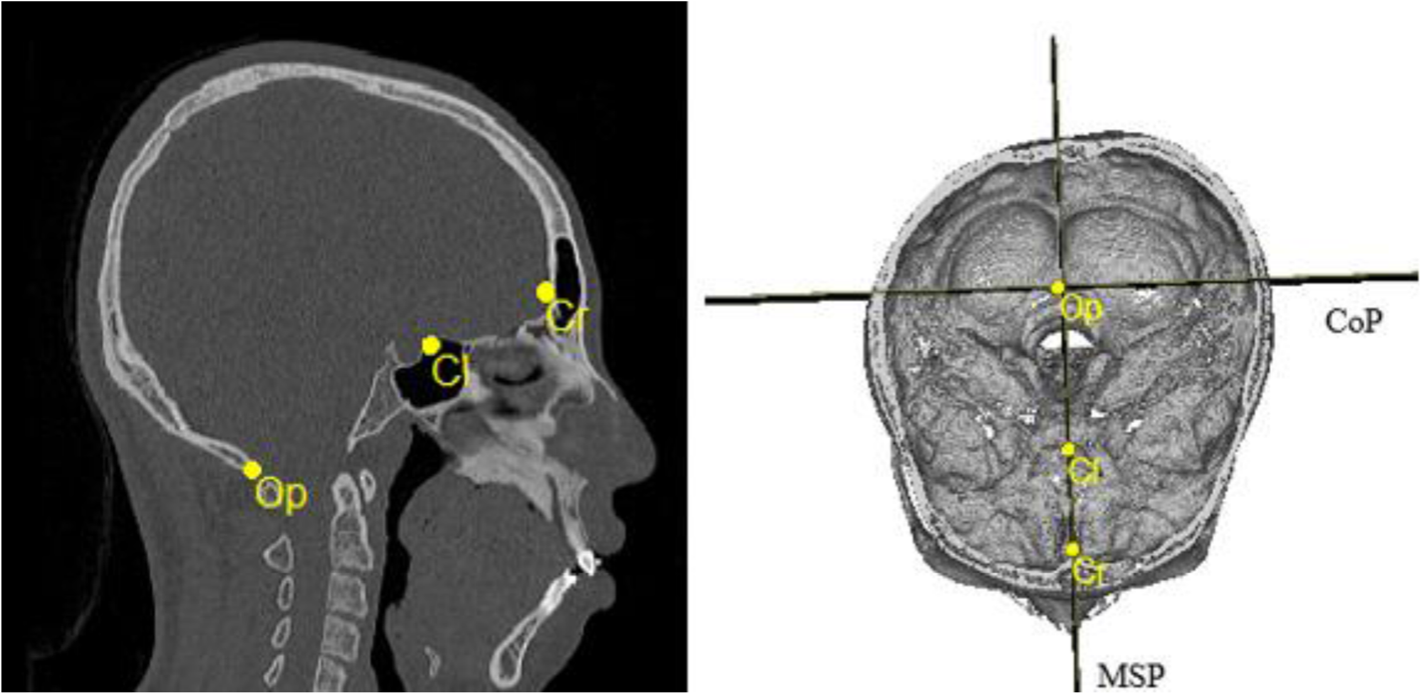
Computed tomography (CT) scans of craniofacial landmarks. Cr, crista galli; Cl, clinoid process; Op, opisthion; MSP, midsagittal plane; CoP, coronal plane
